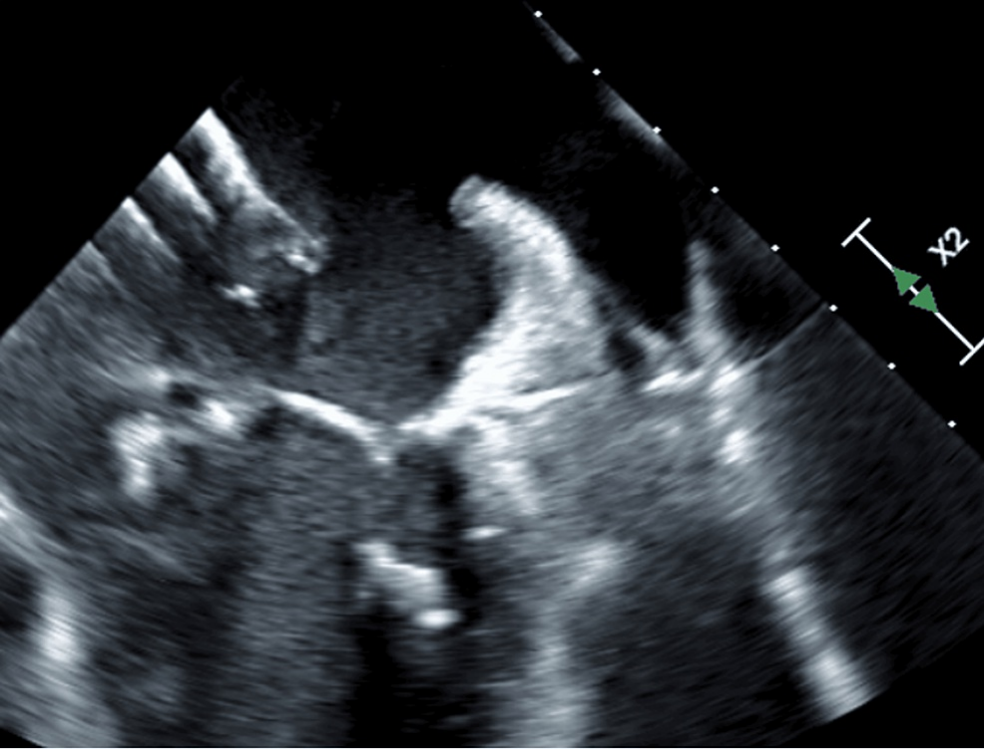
Transesophageal echocardiogram showing Angiovac cannula in the left atrium
